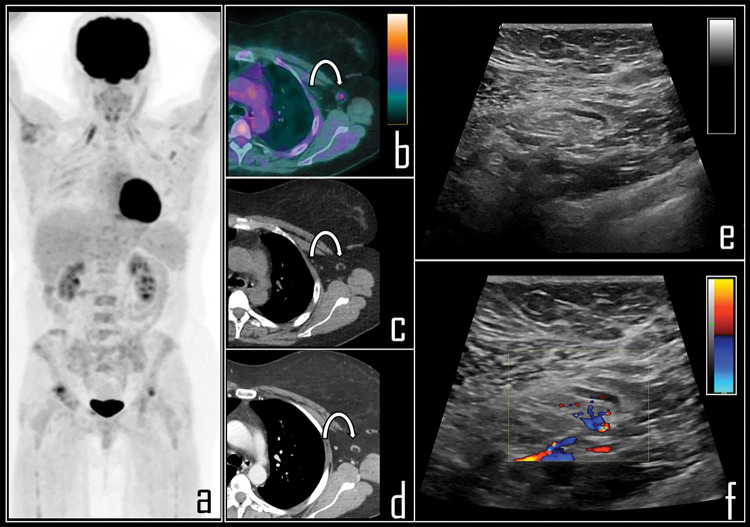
A 65-year-old female patient was examined by means of 2-[^18^F]FDG PET/CT during follow-up of ovarian cancer. PET *Maximum Intensity Projection* (a) was negative for disease relapse but showed a focal area of tracer uptake in the left axilla. Patient was previously submitted (14 days before) to second dose of COVID-19 vaccine in the deltoid muscle of left arm. Axial PET/CT view (b, *curved arrow*) shows the uptake in association with a 0.8 cm wide lymph node, with fatty hilum at correlative *low dose* axial CT (c, *curved arrow*). Subsequently, patient underwent whole body contrast enhanced CT, not showing disease relapse but confirming the axillary lymph node in left axilla, with low contrast enhancement (d, *curved arrow*). Ultrasonography was performed, displaying benign, 0.8 cm wide lymph node with regular shape (e), dominant central hilar flow and peripheral flow at color-doppler (f).
